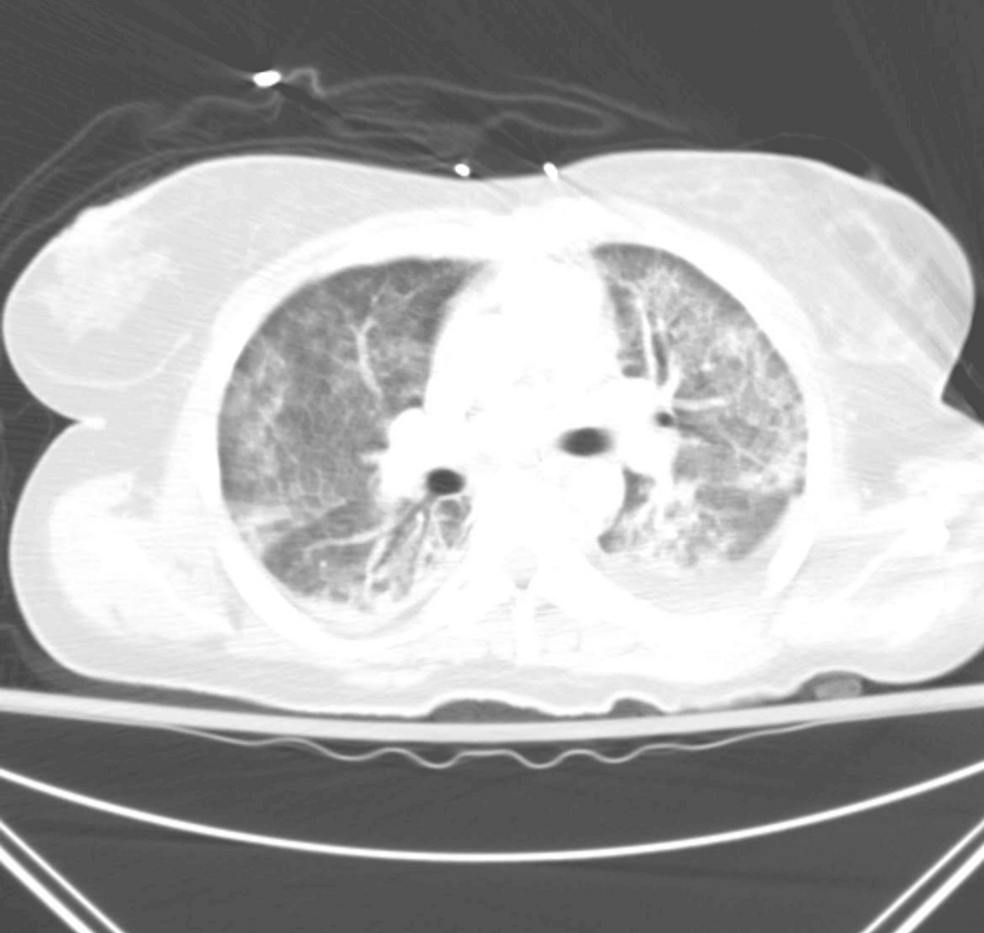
CT angiography of the chest showed bilateral multilobar disease concerning for acute respiratory distress syndrome
